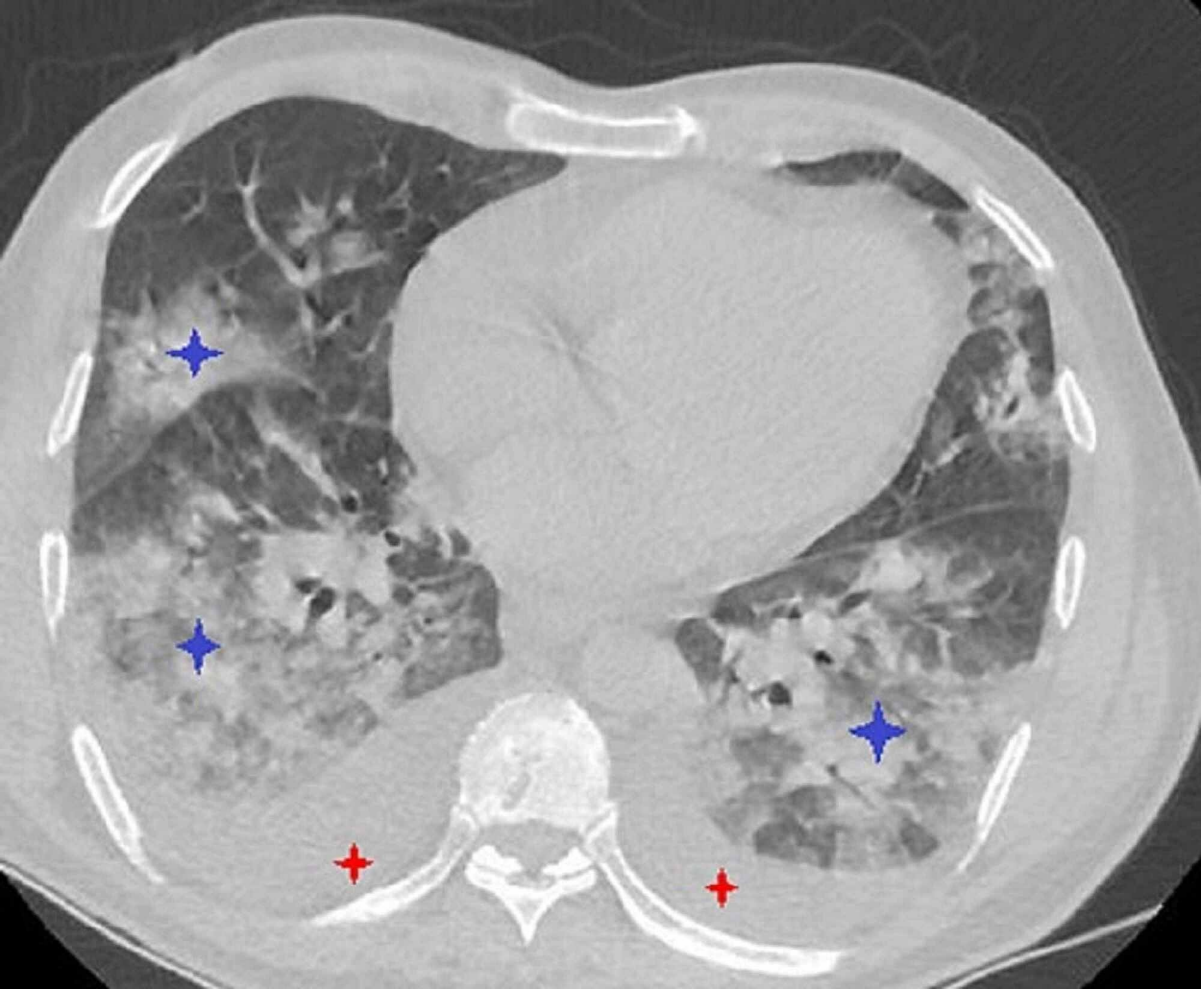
CT of the chest without contrast showing Mild bilateral pleural effusion (red stars); ground-glass opacities with crazy paving aspect predominantly peripheral in distribution (blue stars)
